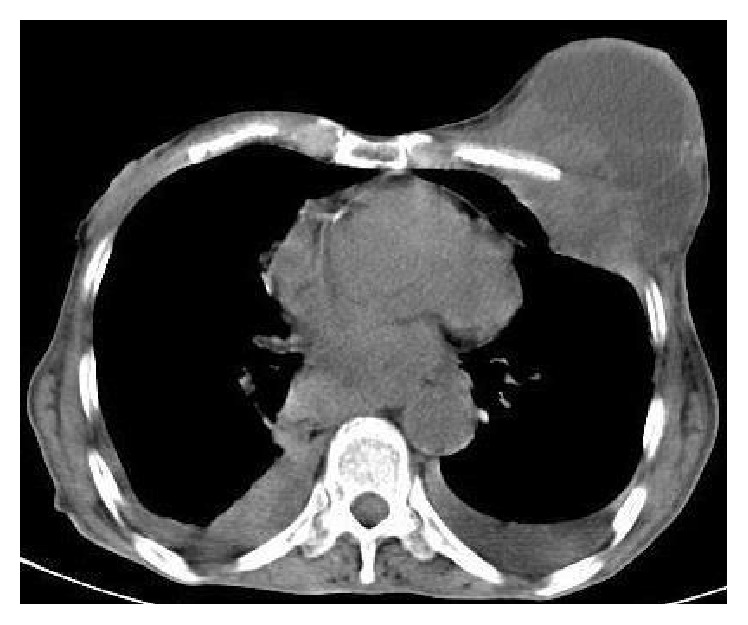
Oral and intravenous contrast-enhanced CT scan of the chest revealed bilateral pleural effusions with breast mass involving the left chest muscles and pleura destroying the rips.
